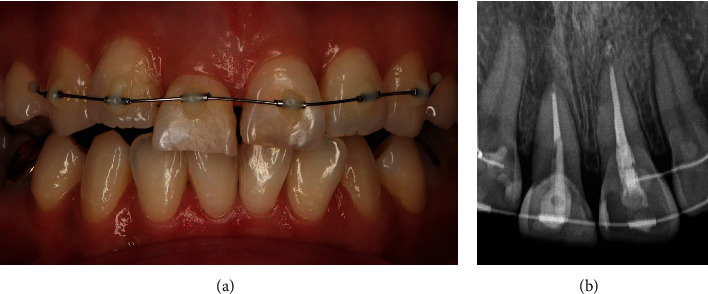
(a) Intra-oral photograph of 4 weeks after orthodontic extrusion started. (b) Periapical view of 4 weeks after orthodontic extrusion started.
